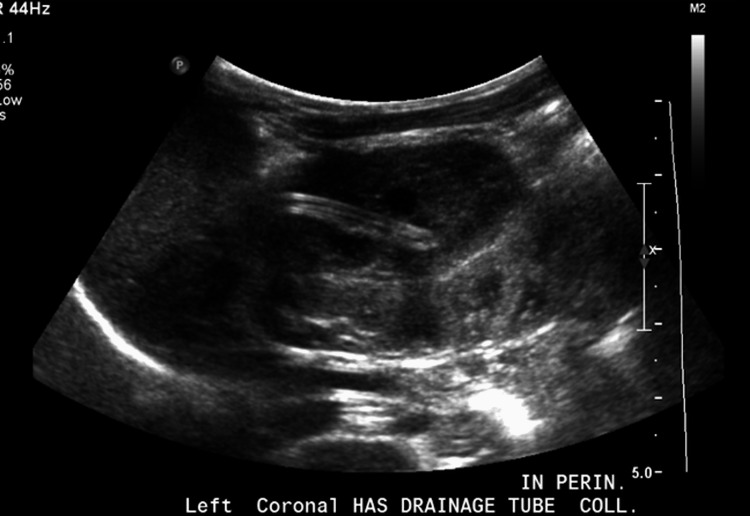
Ultrasound of the left kidney three days after a perinephric drain was placed. Note the decrease in the size of perinephric fluid collection with a drain in situ and decreased compression of the kidney parenchyma.
